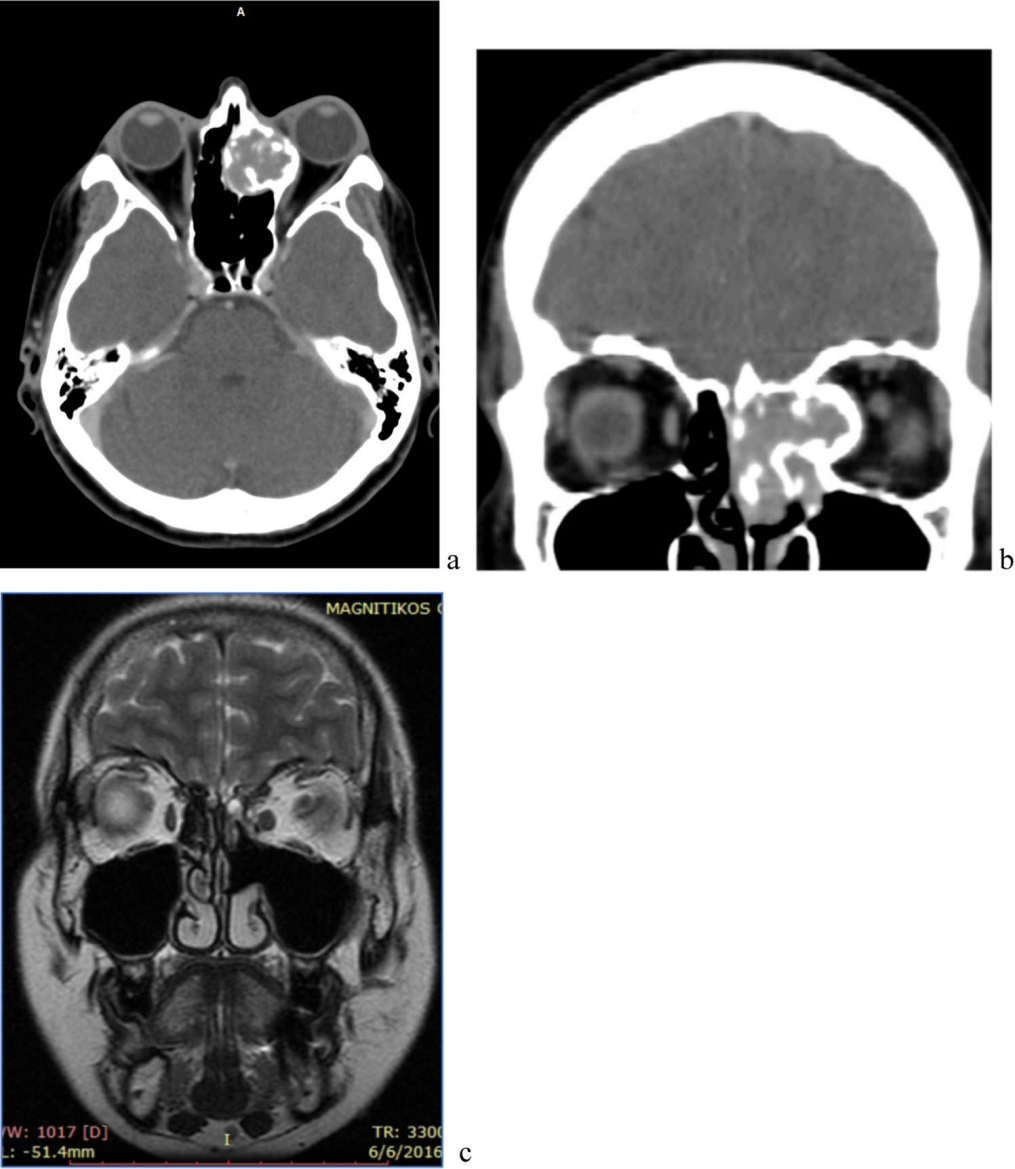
Pre-operative CT scan, axial slice (a) and coronal slice (b) (Case 1), c Post-operative MRI scan (Case 1)
